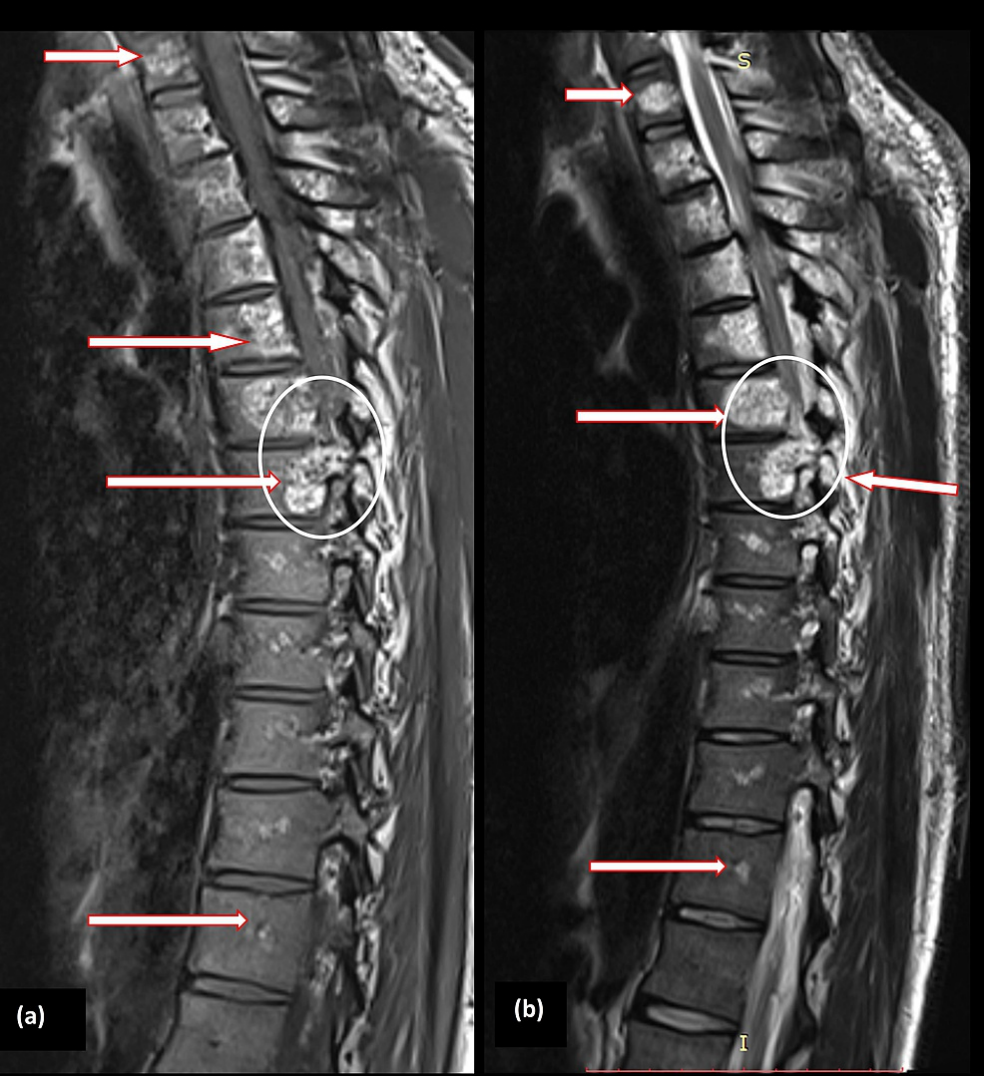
Sagittal views of MRI with contrast showing involvement of 13 vertebrae (marked with arrows in several places in both images (a) and (b)) from C7 to D12 with spinal cord compression (circled).
